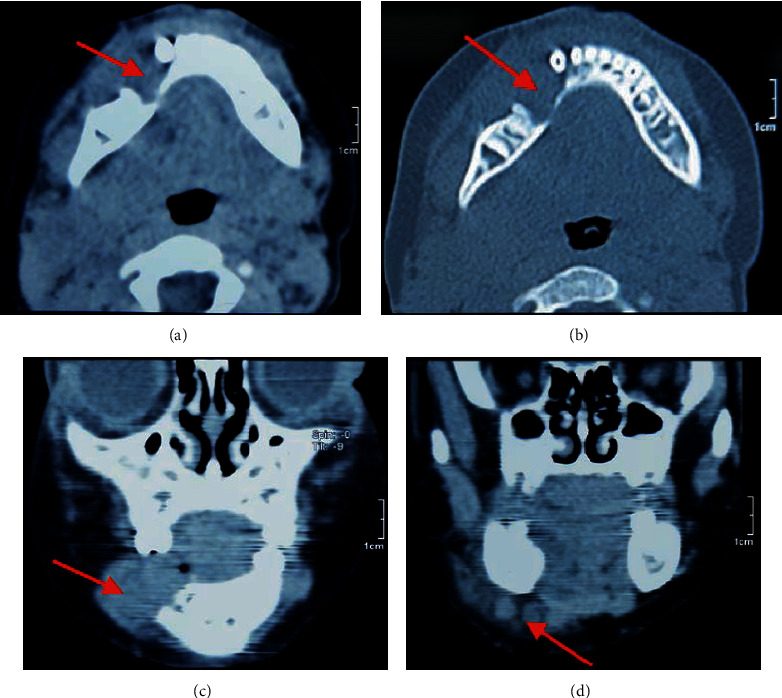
CT images showing a lesion with bony cortex perforation (red arrow, (a) and (b)) and soft tissue infiltration and lymph nodes involvement (red arrow, (c) and (d)).
